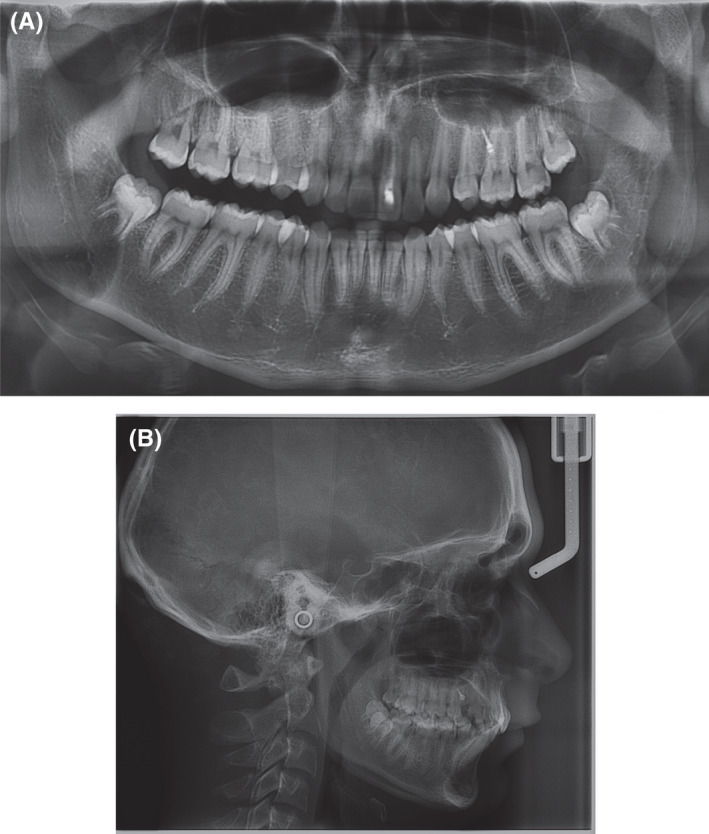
Panoramic and lateral cephalometric radiographs of the patient 4 years post‐operatively in (A) and (B), respectively showing good alignment of the teeth with restoration of normal occlusion
